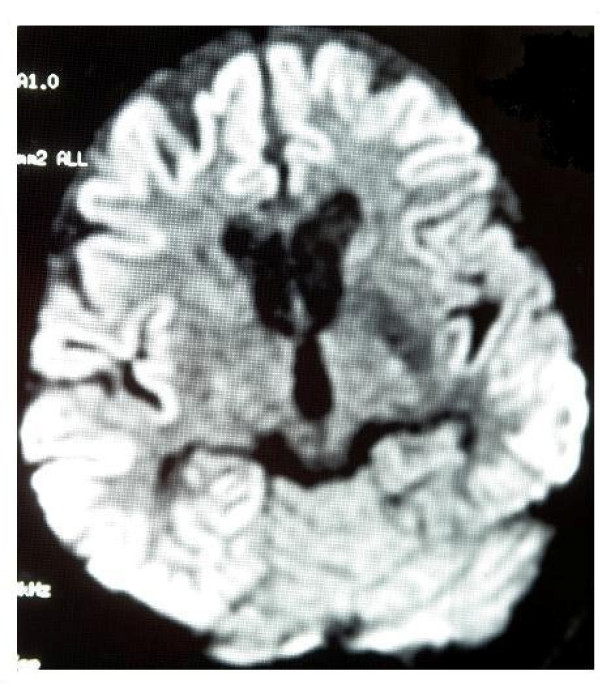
The cortical ribboning sign on diffusion MRI of the patient.
